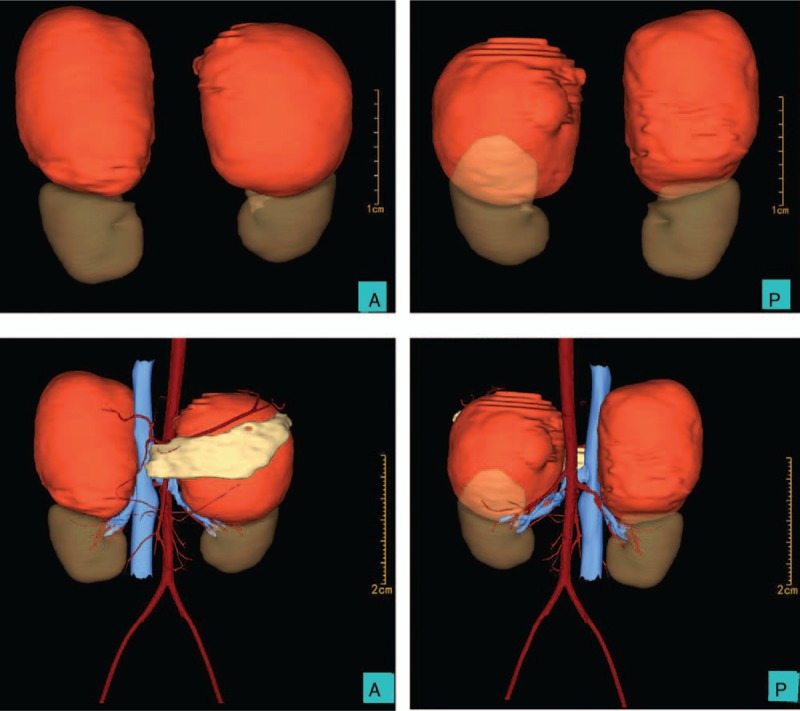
Three-dimensional digital reconstruction of kidneys, blood vessels, and masses based on CT images. Total tumor volume was 1698.6 ml, while the total volume of 2 kindeys was 347.6 ml.
